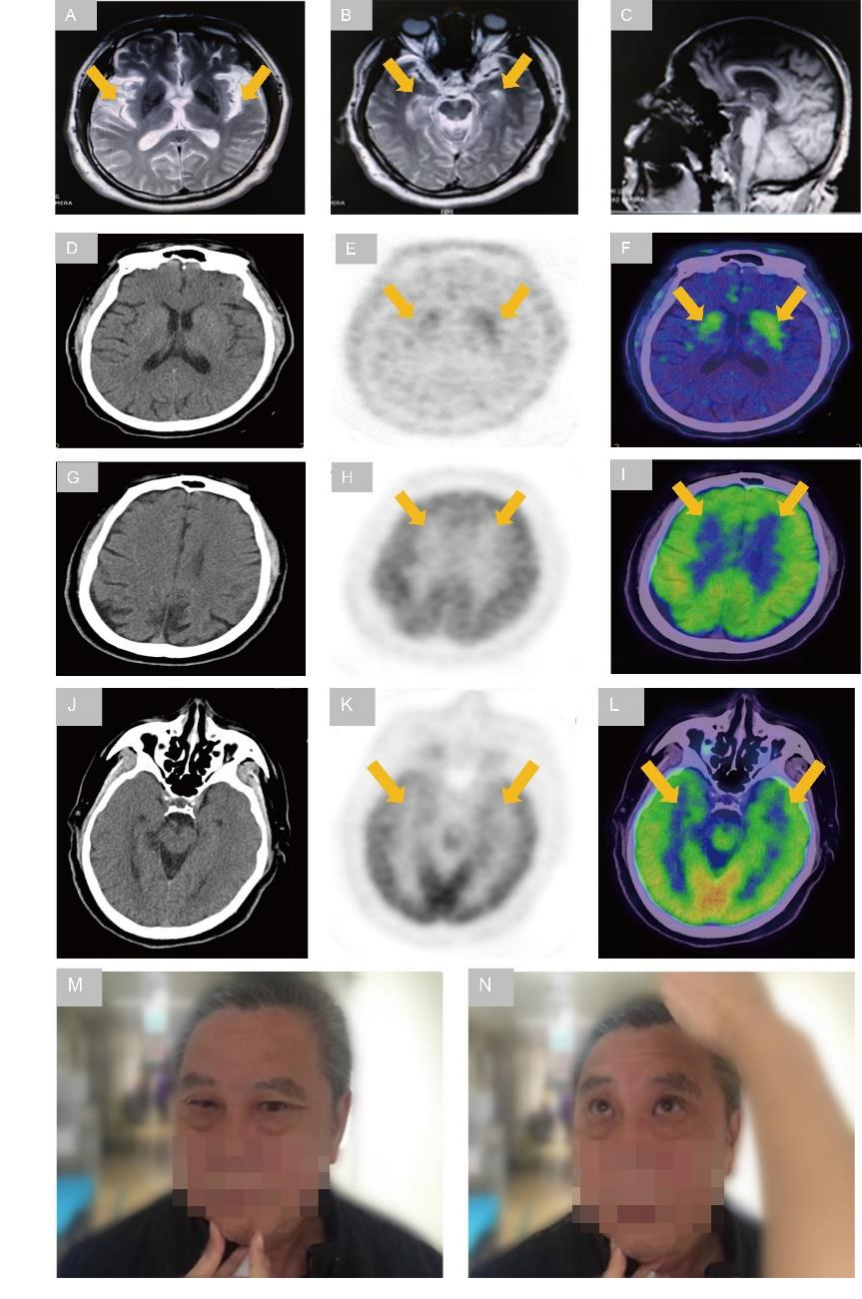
Neuroimaging characteristic of the patient with GRN mutations. (A and B) Axial T2-weighted images showing bilateral lateral fissure deepened and widened (arrow) and dilatation of temporal horn of lateral ventricle (arrow). (C) Sagittal T2-weighted images showing mild generalized cortical atrophy. (D-F) ^18^F-DOPA PET/CT scans showing profoundly reduced DOPA metabolism in bilateral corpus striatum (arrow). (G-I)^18^F-FDG PET/CT scans showing a symmetrically reduced glucose metabolism in bilateral frontal lobe (arrow). (J-L) ^18^F-FDG PET/CT scans showing a symmetrically reduced glucose metabolism in bilateral temporal lobe (arrow). (M) This photograph showing the impossibility of the patient to look downward with the guidance of a neurologist. (N) This photograph showing the difficulty of the patient to look upward with the guidance of a neurologist.
